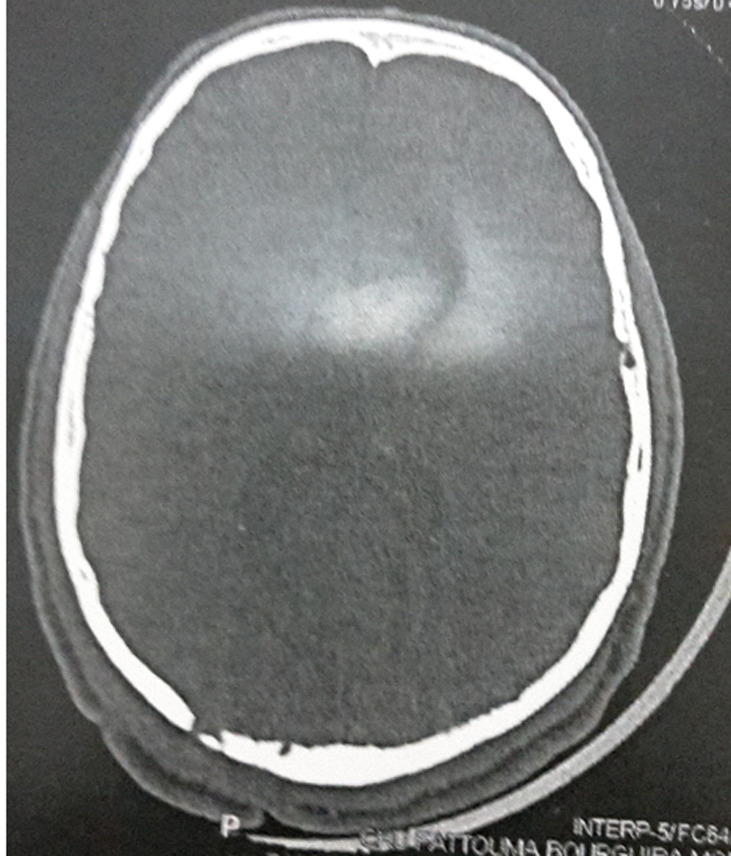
CT-scan osteolysis of the skull in the occipital region near to the primary lesion
